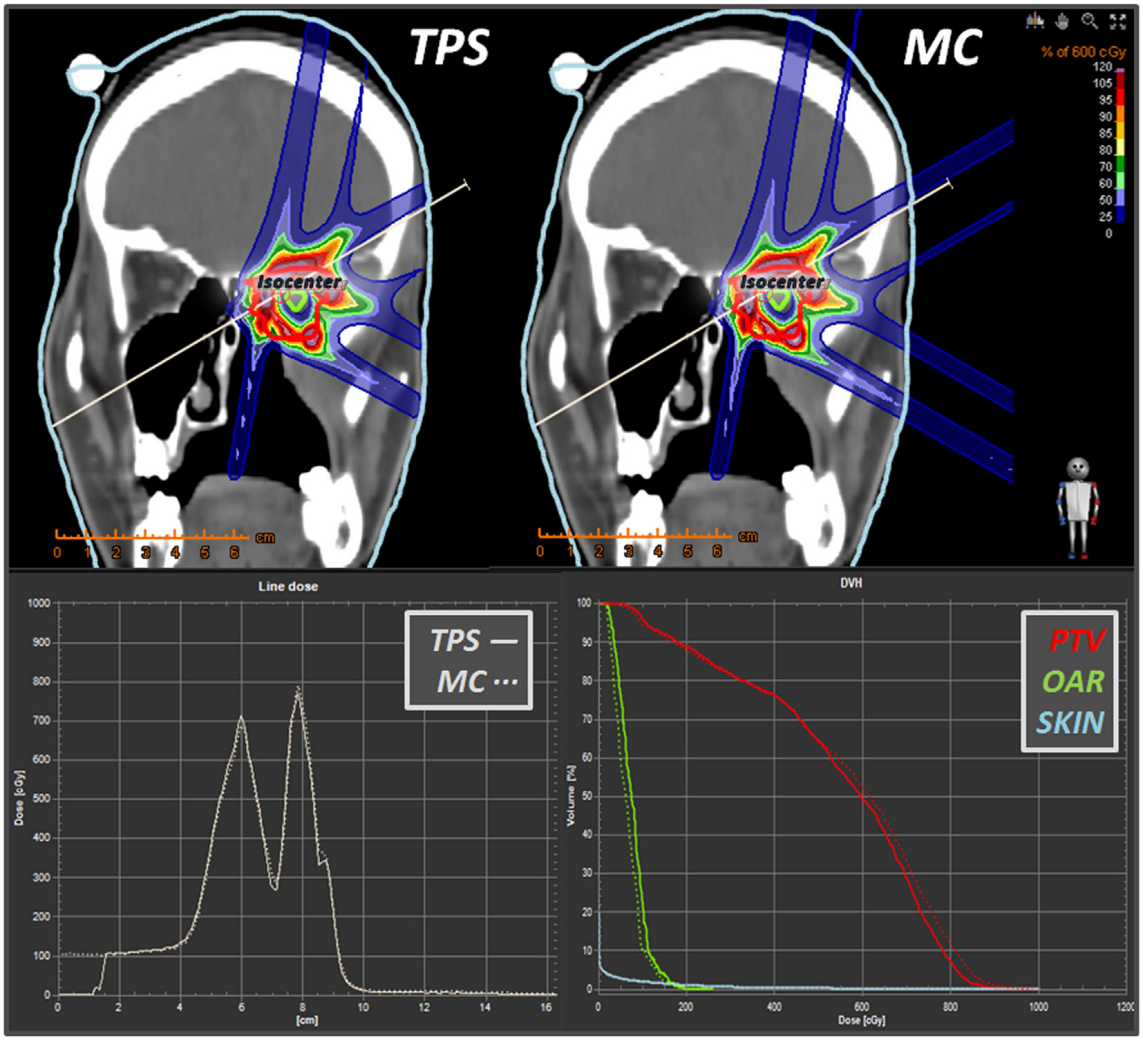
Some snapshots taken from RayStation^®^ showing a comparison (in coronal view) between a *syngo*^®^ 3-fields carbon ions plan vs. its MC dose forward recalculation. Lower panels display the line dose and the DVH calculator tools. Differently from the TPS, which displays dose only within the “external-type” structure, the MC dose-to-water scoring is extended to the whole field of view of the CT scan.
